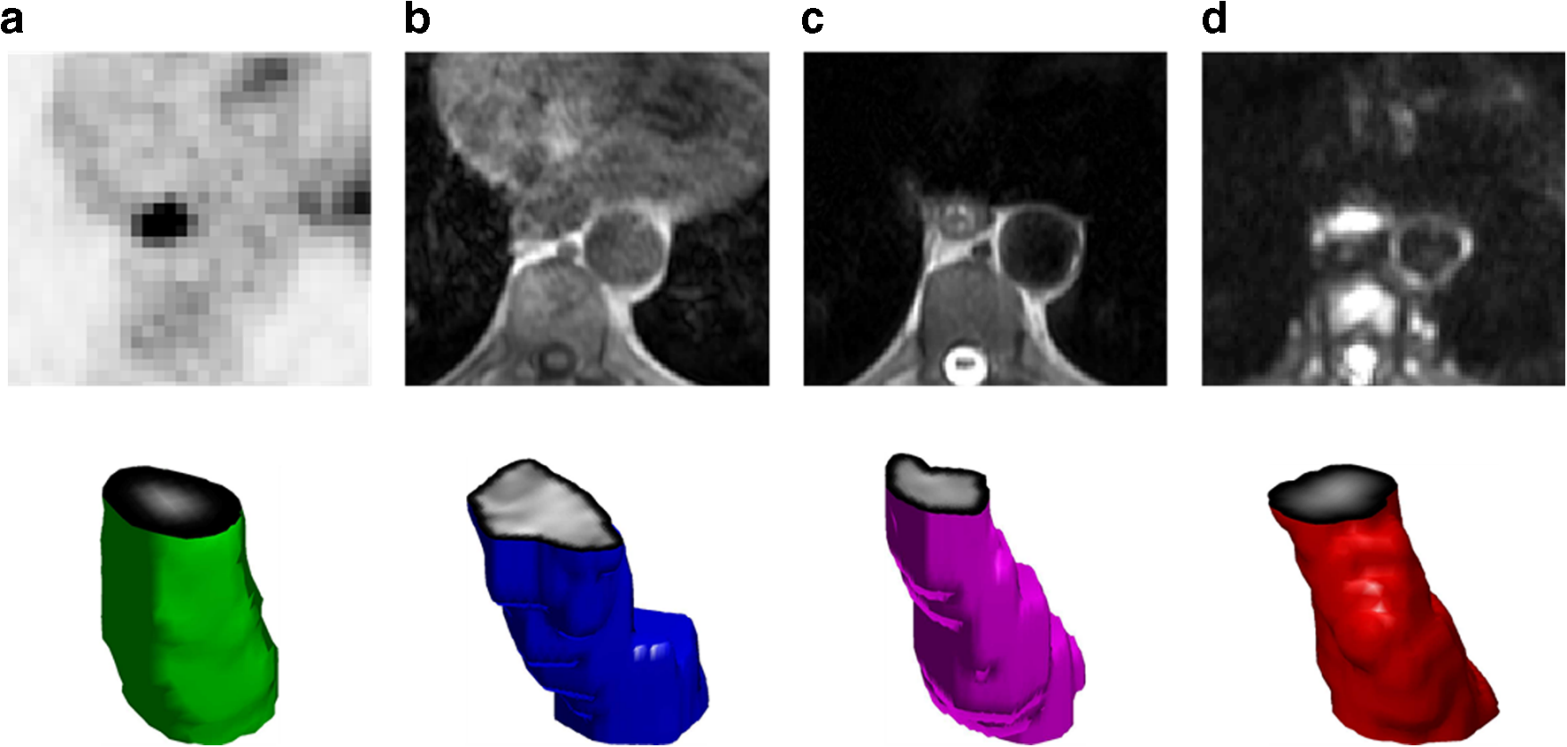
Axial ^18^F-FDG PET (a), T1-weighted (b), T2-weighted (c) and diffusion weighted (d) axial images of a GOJ cancer and respective tumour volumes
